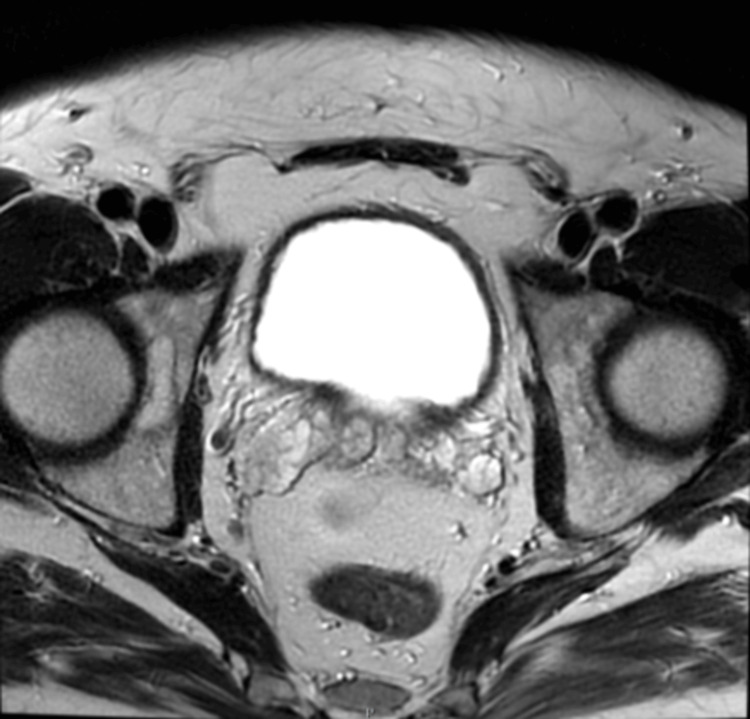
MRI image (axial T2) showing asymmetry of the seminal vesicles and an enlarged right seminal vesicle at level of vesico-ureteric junction MRI, magnetic resonance imaging
